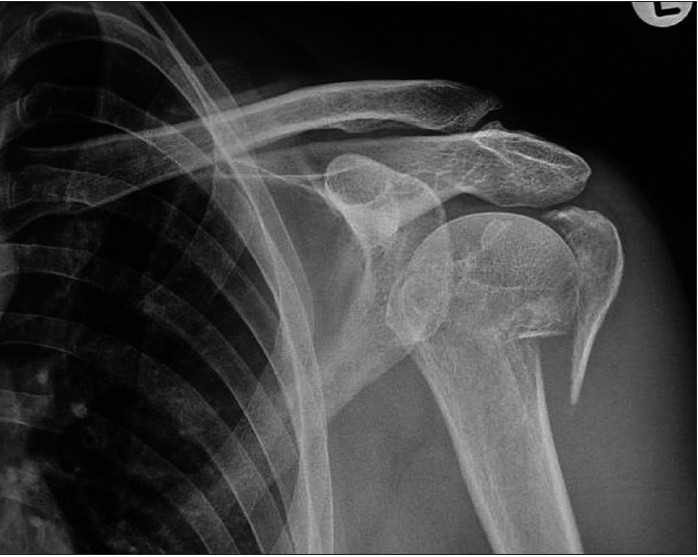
Preoperative anteroposterior radiograph of a 54-year-old female with a four-part fracture in her left proximal humerus
